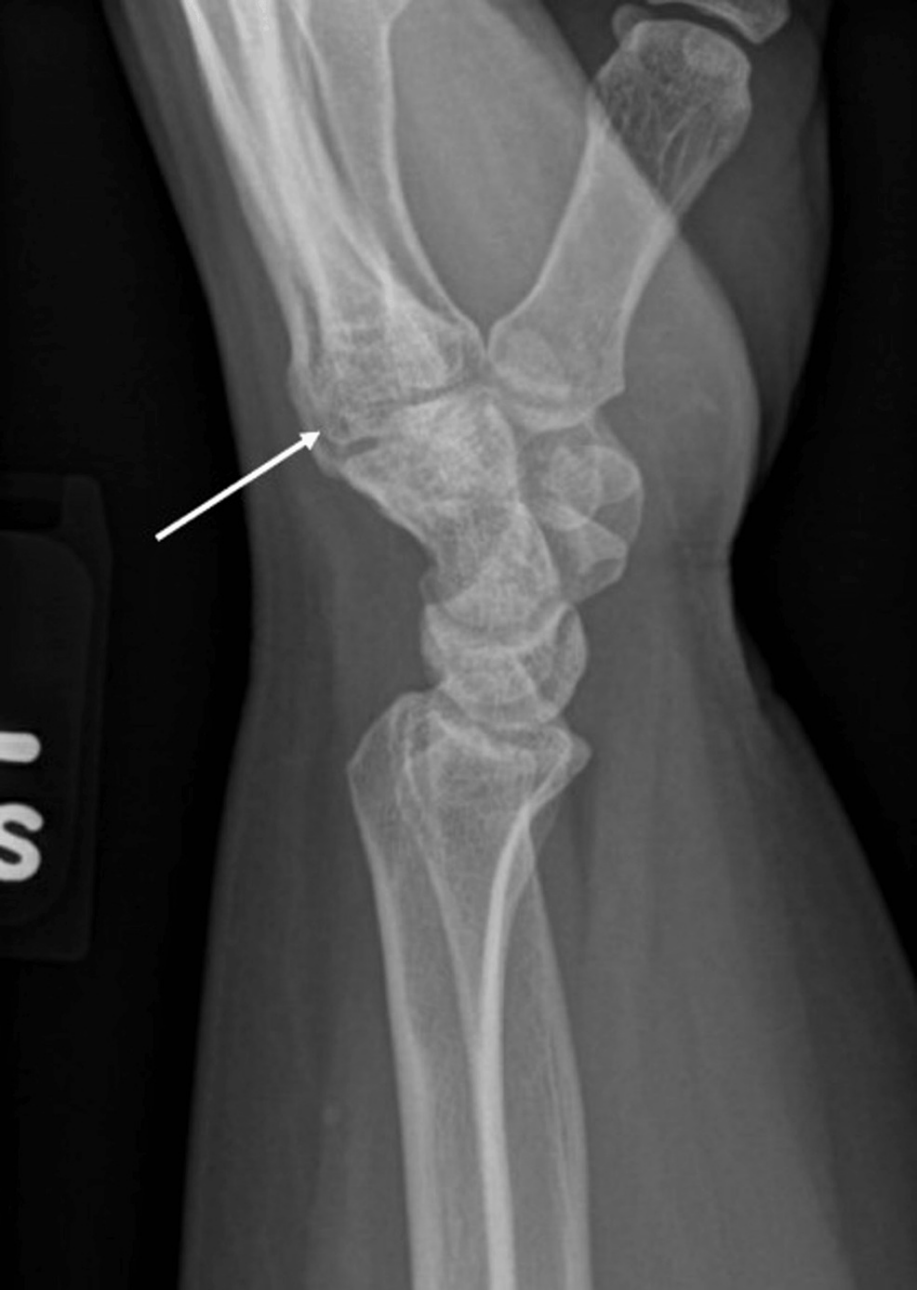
Pre-operative lateral radiograph of the left hand.
